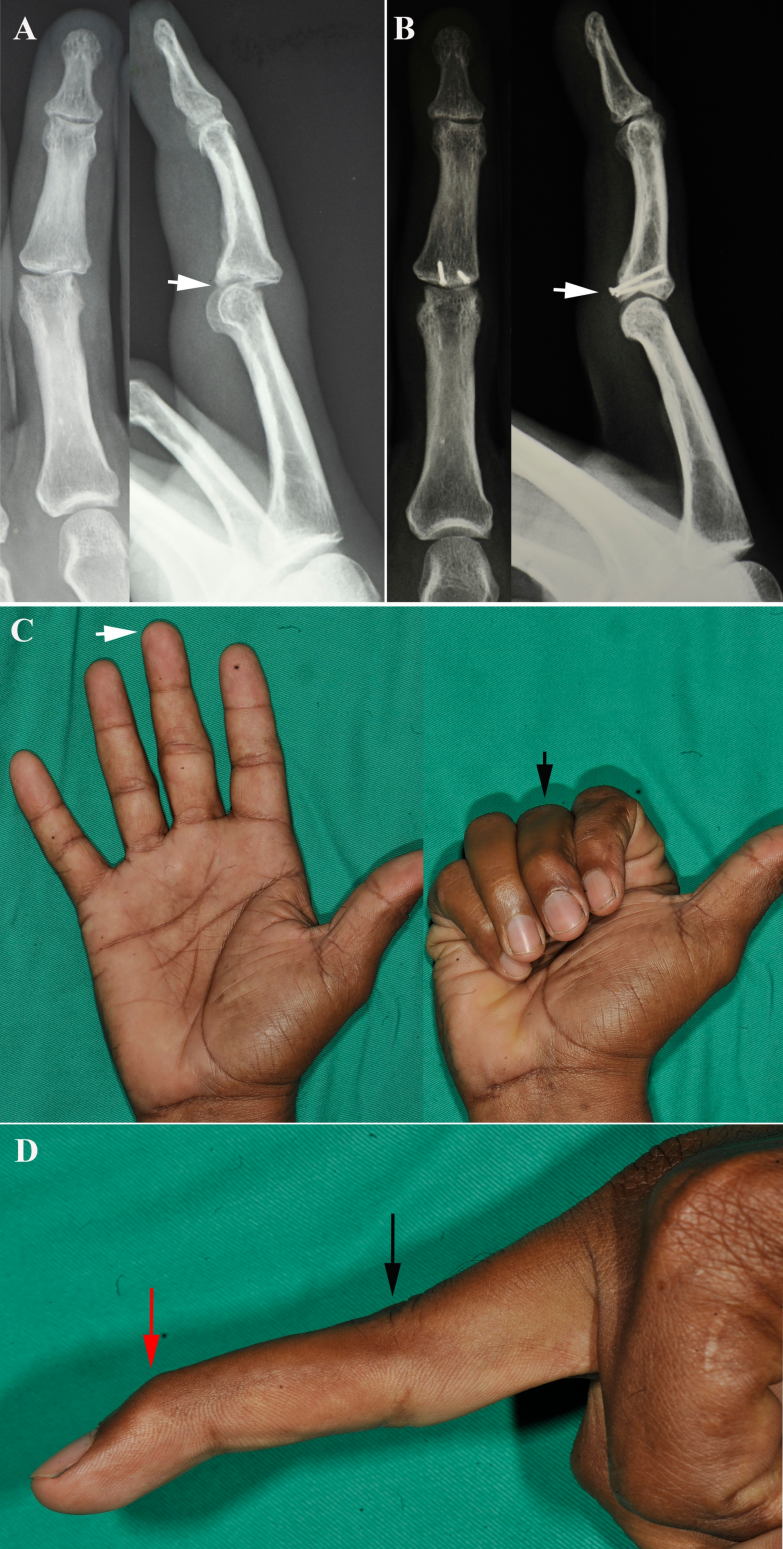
Case Report Two (A) Preoperative radiographs showed malunited base of middle phalanx right middle finger with dorsal subluxation of proximal interphalangeal joint (white arrow). (B) Postoperative radiographs at 24 months follow-up revealed graft union with no joint subluxation (white arrow). (C) At 24 months follow-up, good functional range of motion of the right middle finger in extension (white arrow) and flexion (black arrow) despite swan neck deformity of the finger, where the proximal interphalangeal joint was hyperextended (black arrow) and distal interphalangeal joint was flexed (red arrow) (D).
